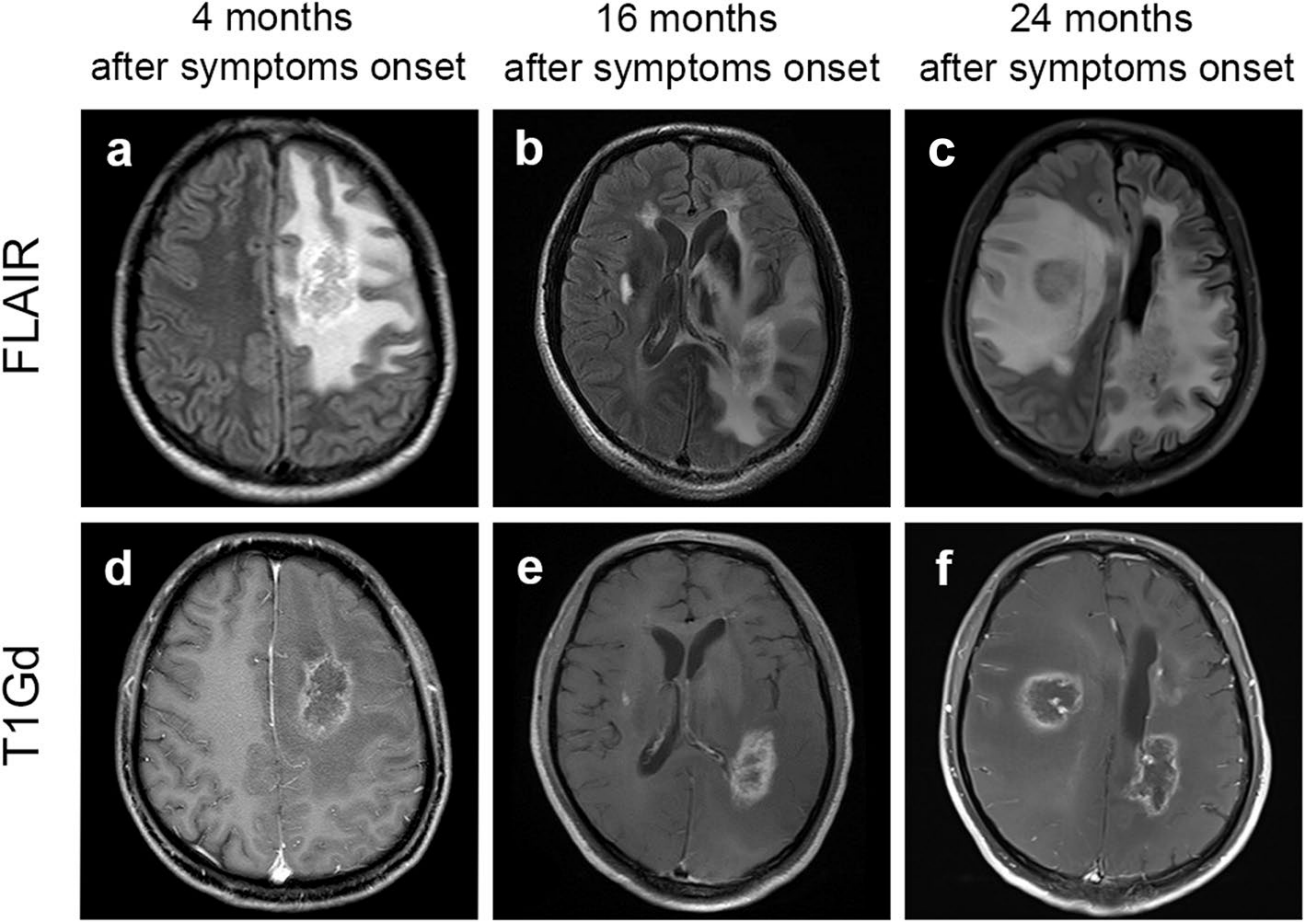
Serial brain magnetic resonance images (MRIs) of the patient during two years. Fluid attenuated inversion recovery (FLAIR) and T1-weighted gadolinium-enhanced (T1Gd) axial images were acquired at 4 (a, d), 16 (b, e), and 24 (c, f) months after symptoms onset. Images were taken 4 months after symptoms onset (a, d) and showed a closed-ring enhanced lesion with perilesional edema. Images acquired at month 16 (b, e) and 24 (c, f) revealed new lesions in the left temporal-occipital lobe and right temporal lobe. The right one progressed from a nodular enhancing lesion to a rim-enhancing lesion with edema
